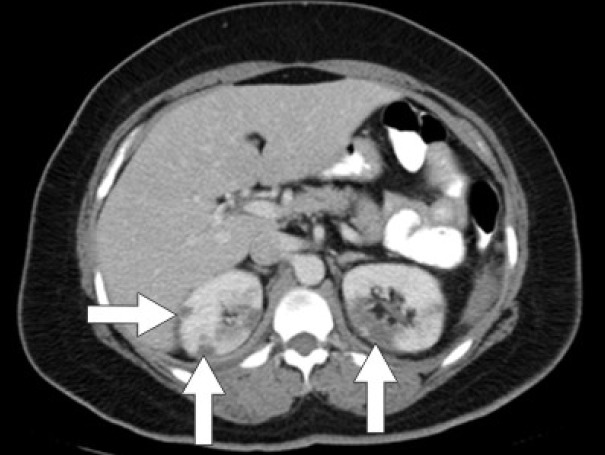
CT scan of the abdomen showing wedge-shaped hypodensities in both kidneys bilaterally consistent with renal infarcts.
